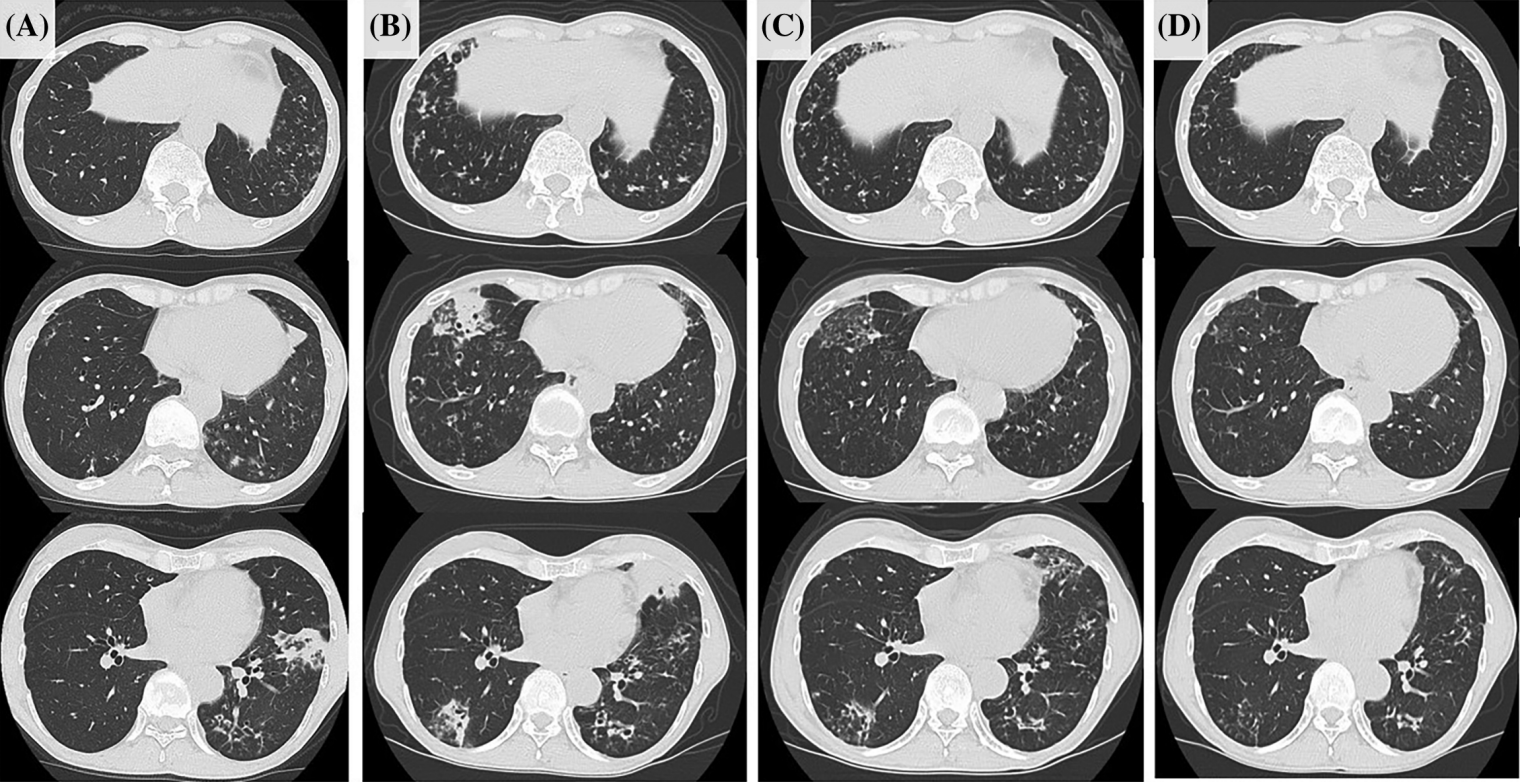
(A) Chest computed tomography (CT) revealed bronchiectasis and a small nodular shadow along the airways in both lungs at the first visit. (B) Chest CT before introduction of ceftazidime (CAZ) shows multiple nodules and consolidations in addition to a gradual exacerbation of the bronchiectasis and small nodular shadow along the airway in both lungs, which were detected 3 years ago. (C) Four weeks after the administration of CAZ, the nodular and infiltrative shadows decreased. (D) Two and a half months later, these findings had further improved.
